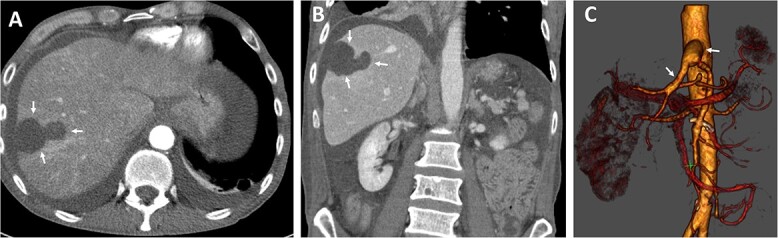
Postoperative axial (A) and coronal (B) CT angiographic images demonstrate enhancement during contrast-enhanced phase and hepatic ischemic area with irregular margins. (C) 3D reconstructed CT angiography shows antegrade visceral reconstruction with a bifurcated graft from the supra-celiac aortic donor to the superior mesenteric and celiac arteries (arrows).
